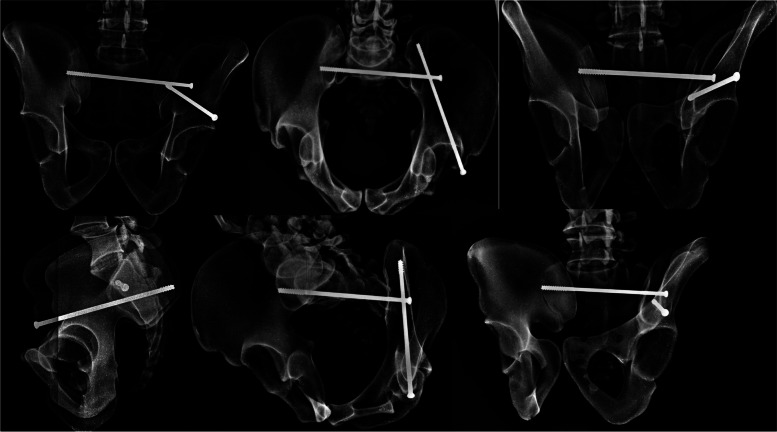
AP, Inlet, Outlet, Lateral, Obturator Inlet and Obturator Outlet X-Rays
